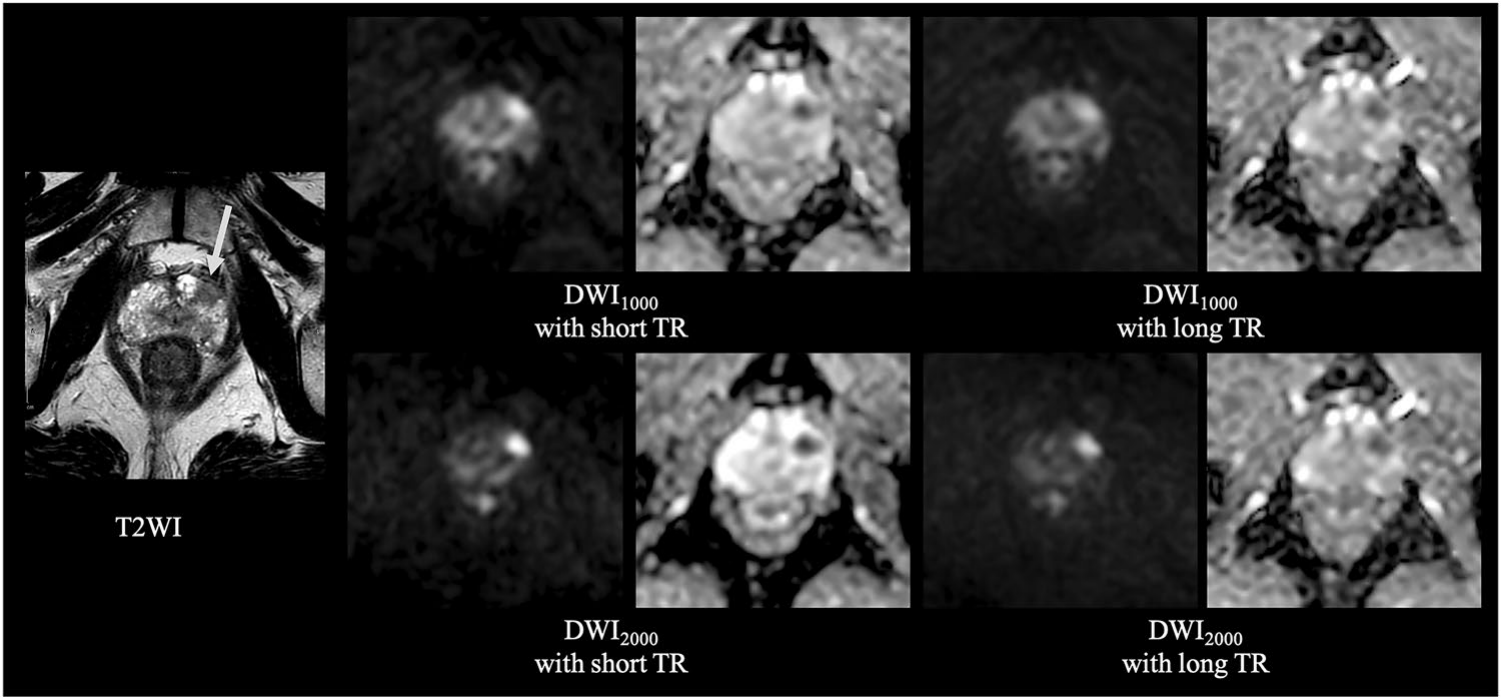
Representative images for T2WI, DWI at a b-value of 1000 s/mm^2^ (DWI_1000_) with short and long TR (upper row), and DWI at a b-value of 2000s/mm^2^ (DWI_2000_) with short and long TR (lower row). Images in a 63-year-old man with Gleason score 4 + 5 PCa (T2c; prostate-specific antigen [PSA]: 4.8 ng/mL). Transverse T2WI shows a low signal intensity lesion in the left peripheral zone (white arrow). DWI_1000_ with short TR shows better lesion contrast than DWI_1000_ with long TR, whereas DWI_2000_ with short TR shows comparable image quality compared to DWI_2000_ with long TR. DWI; diffusion-weighted imaging, TR; repetition time
